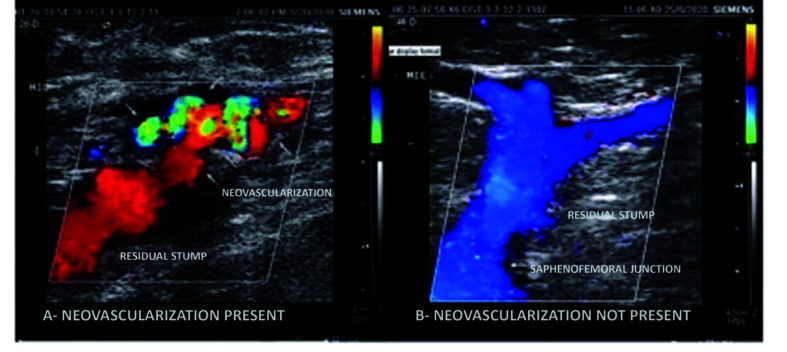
Analysis with color Doppler ultrasonography of the saphenofemoral junction.
